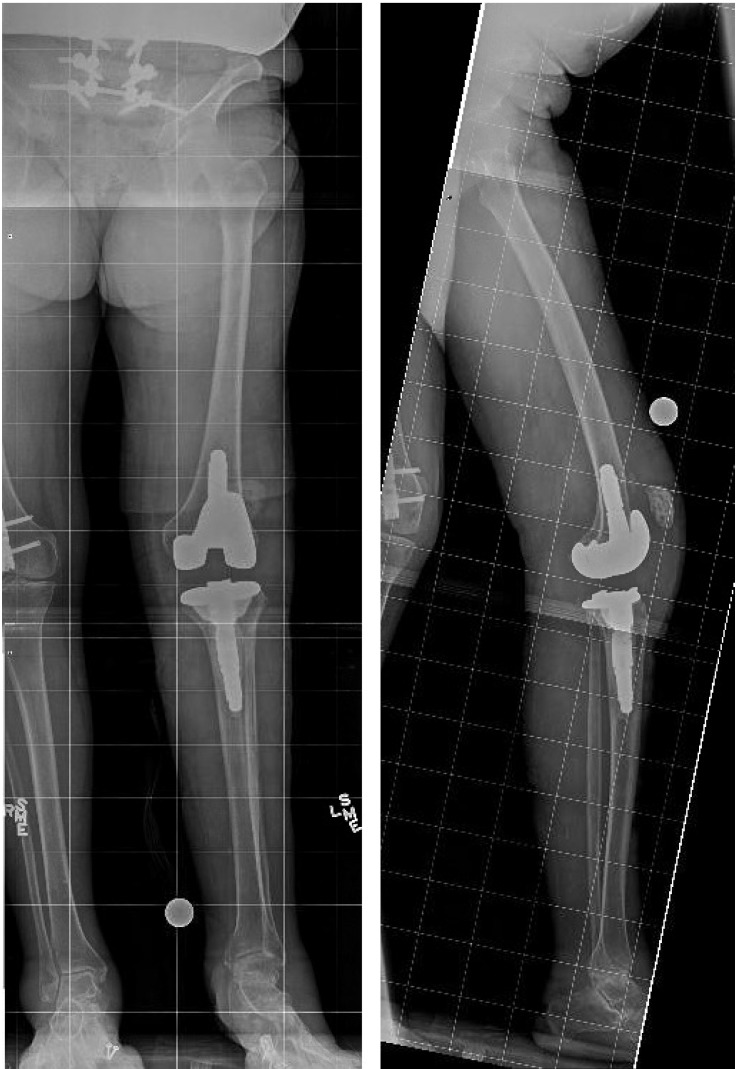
7/30/2013. AP (A) and Lateral (B) radiographs at the time of initial consultation demonstrating a stemmed TKA with substantial effusion and patella alta.
